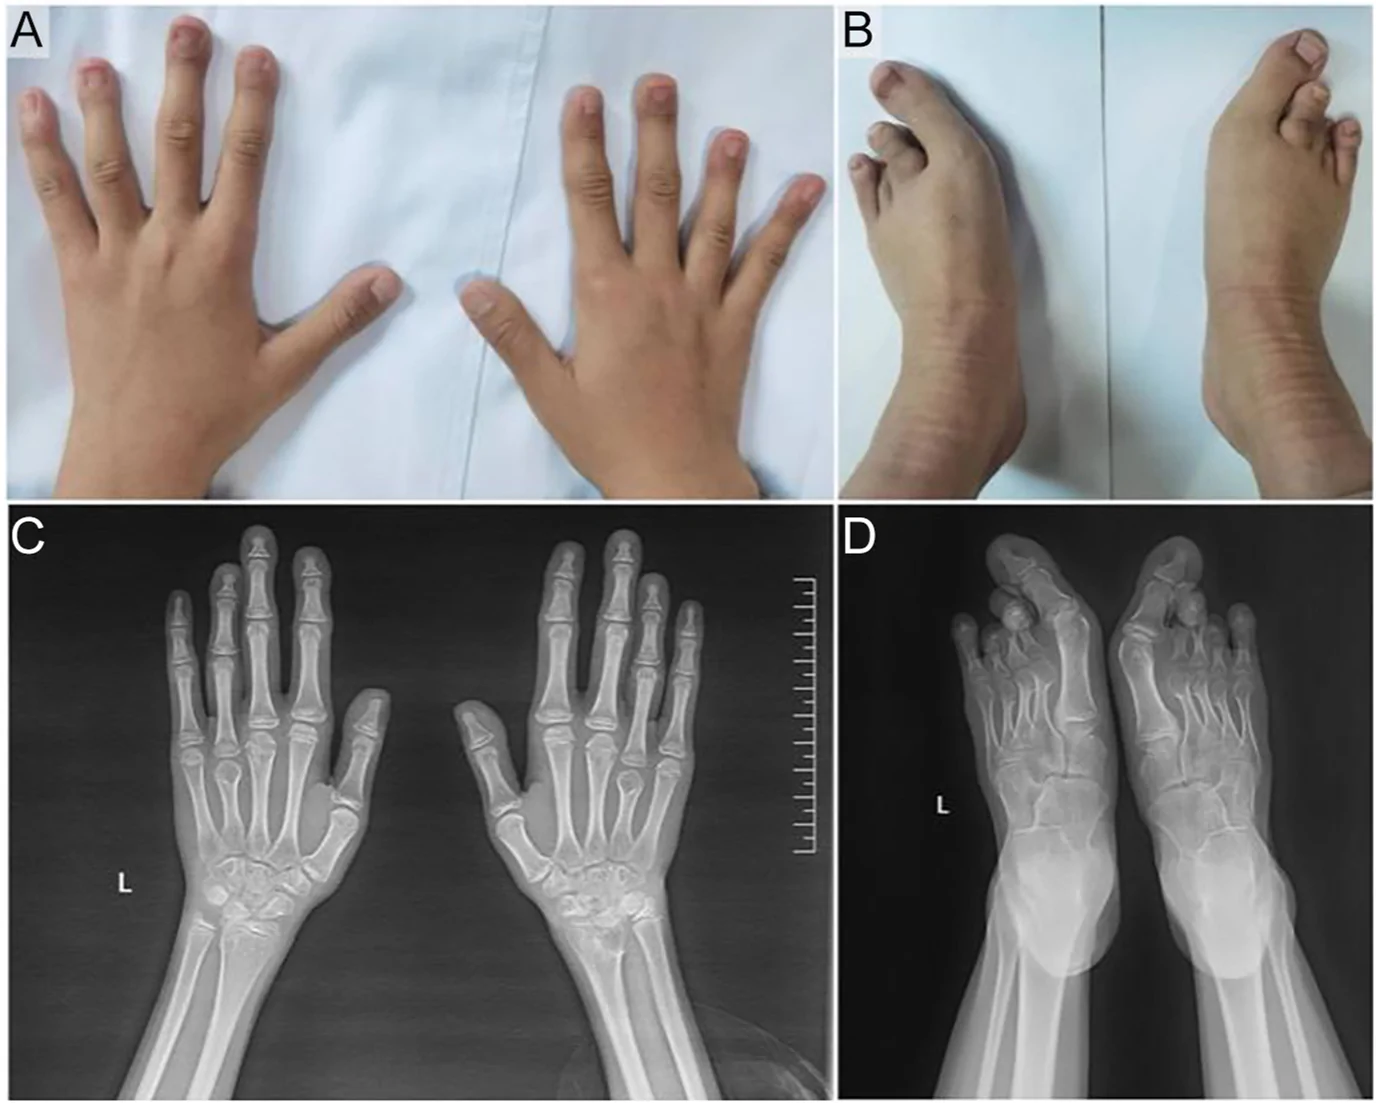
Clinical and radiographic skeletal findings in case 1. Clinical photographs showing shortening of the fingers (A) and toes (B). Radiographs confirmed shortening of the metacarpal bones of both hands (C) and metatarsal bones of both feet (D).
